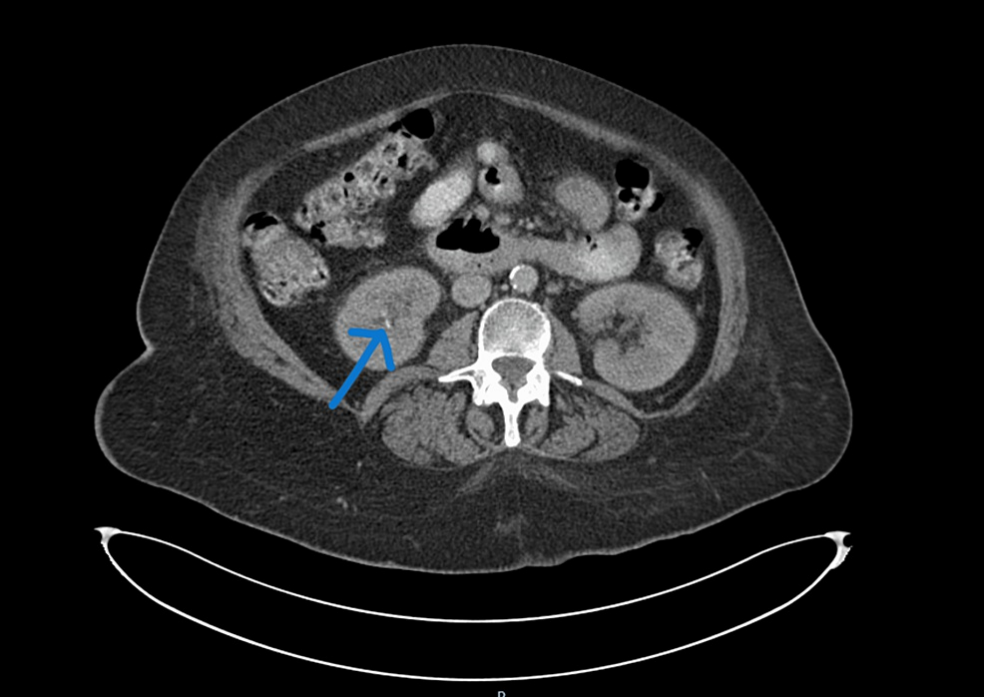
Axial view of the 4mm non-obstructing stone in the right kidney on the CT scan
